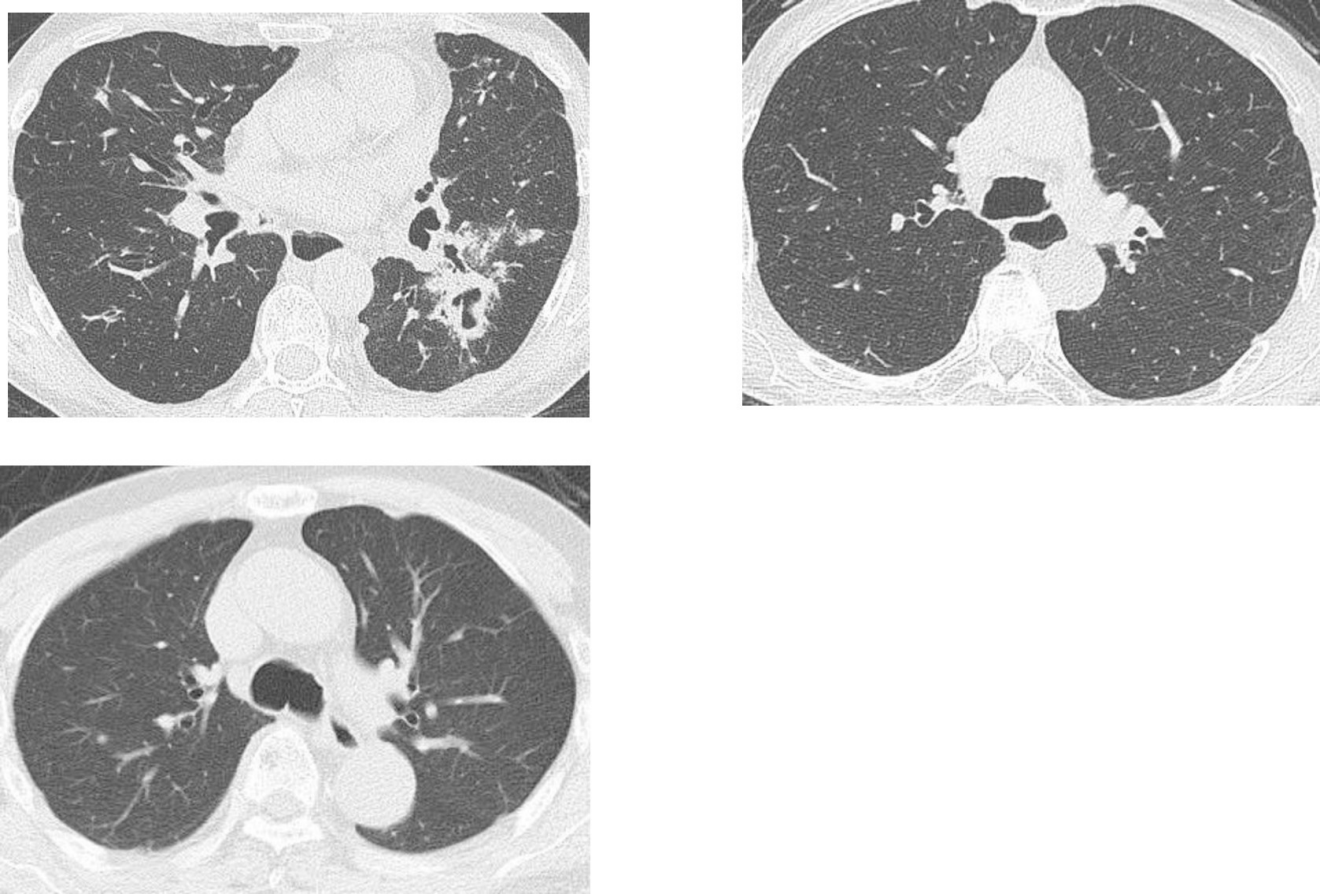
Typical cases of esophageal dilatation. CT images of three cases whose maximum diameter of esophagus is larger than 15mm were shown.
